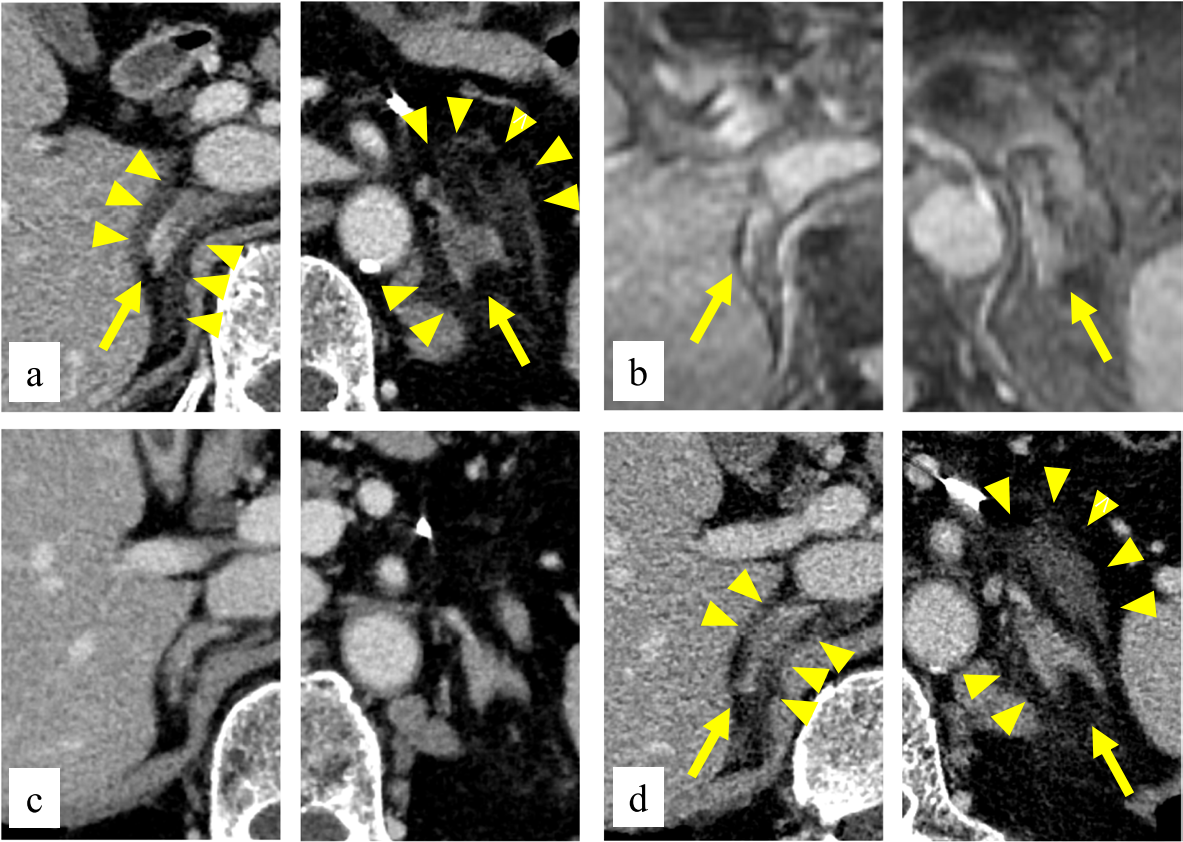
a Contrast-enhanced CT on day 1 showed bilateral adrenal hypertrophy (arrows), non-contrast-enhancing areas, and adjacent fatty inflammatory changes (arrowheads). Capsular signs were also observed. b No adrenal hemorrhage evidence was observed in T2 star-weighted MRI images on day 5 (arrows). c Contrast-enhanced CT on day 9 showed improvement in bilateral adrenal hypertrophy, non-contrast-enhancing areas, and adjacent fatty inflammatory changes. d Contrast-enhanced CT on day 62 demonstrated the same findings as on day 1, revealing bilateral adrenal infarction recurrence
